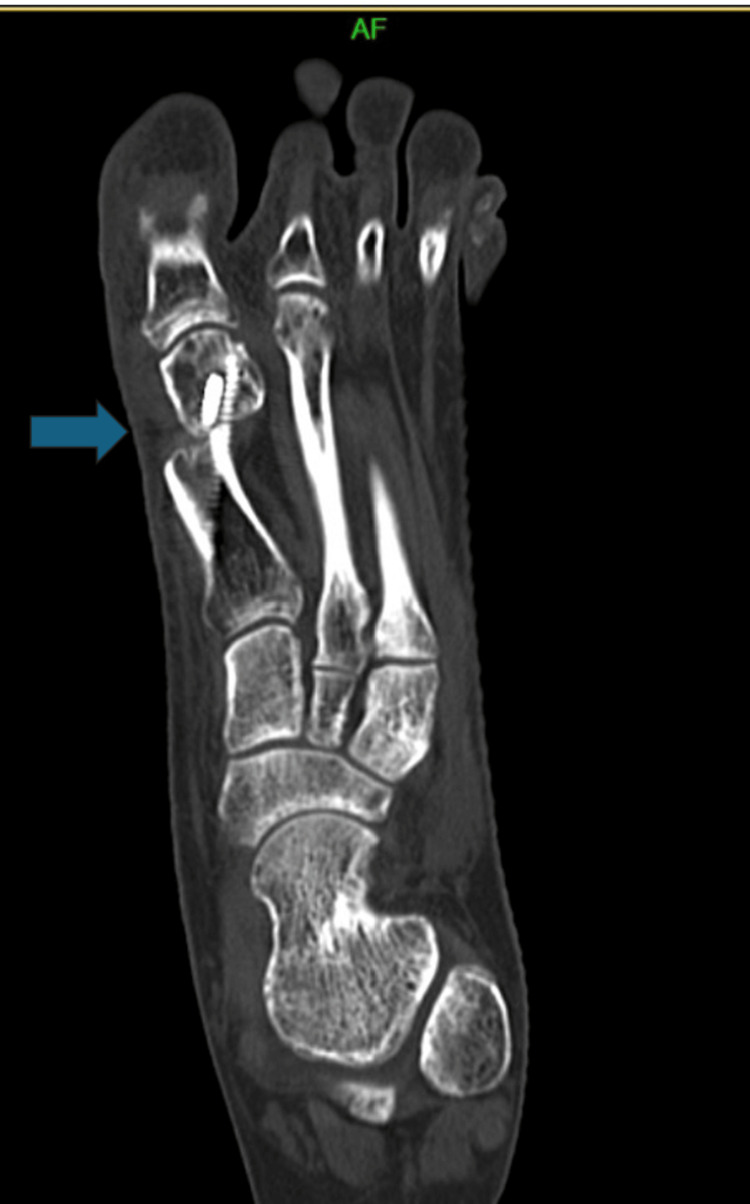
Coronal CT image confirming absence of osseous union across the osteotomy site (blue arrow), indicating the persistent gap at the osteotomy margins
